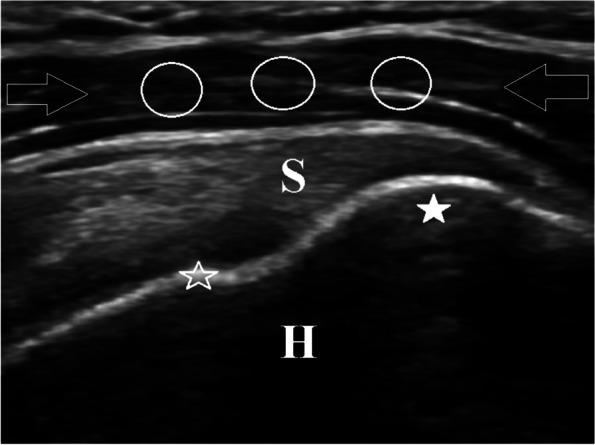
Ultrasound of a 68-year-old man without type 2 diabetes mellitus or obesity. This long-axis sonographic image of the left deltoid muscle (open arrows) is obtained at the anterior aspect of the supraspinatus tendon (S), at its insertion at the greater tuberosity (solid star) of the proximal humerus (H). The 3 circles overlying the deltoid muscle indicate the location of the grayscale pixel intensity region of interest measurements that were obtained to calculate the mean deltoid muscle value. The open star indicates the location of the single region of interest measurement obtained on the osseous cortex of the humeral head, near the anatomic neck. Notice the hypoechoic appearance of the deltoid muscle. The patient had a body mass index of 24 kg/m2. The calculated ratio (deltoid muscle/humeral cortex) for this patient was equal to 0.29, consistent with a non-type 2 diabetes mellitus status
